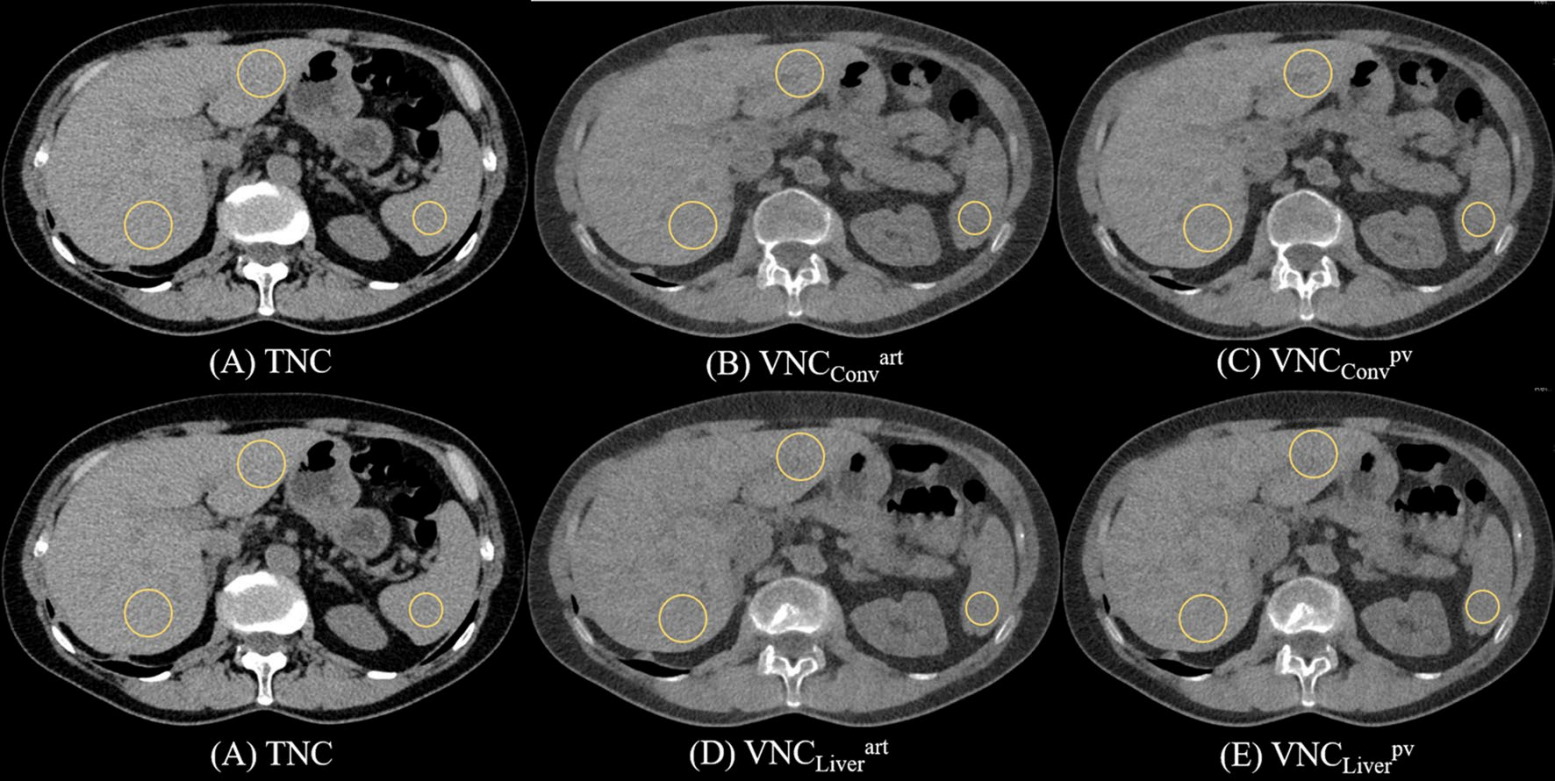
TNC and VNC Images. Note: True non-contrast CT scan (A) with corresponding virtual non-contrast images derived from arterial phase and reconstructed with VNC_conv_ (B) and VNC_Liver_ algorithm (C). Images from portal venous phase and reconstructed with VNC_conv_ (D) and VNC_Liver_ algorithm (E)
